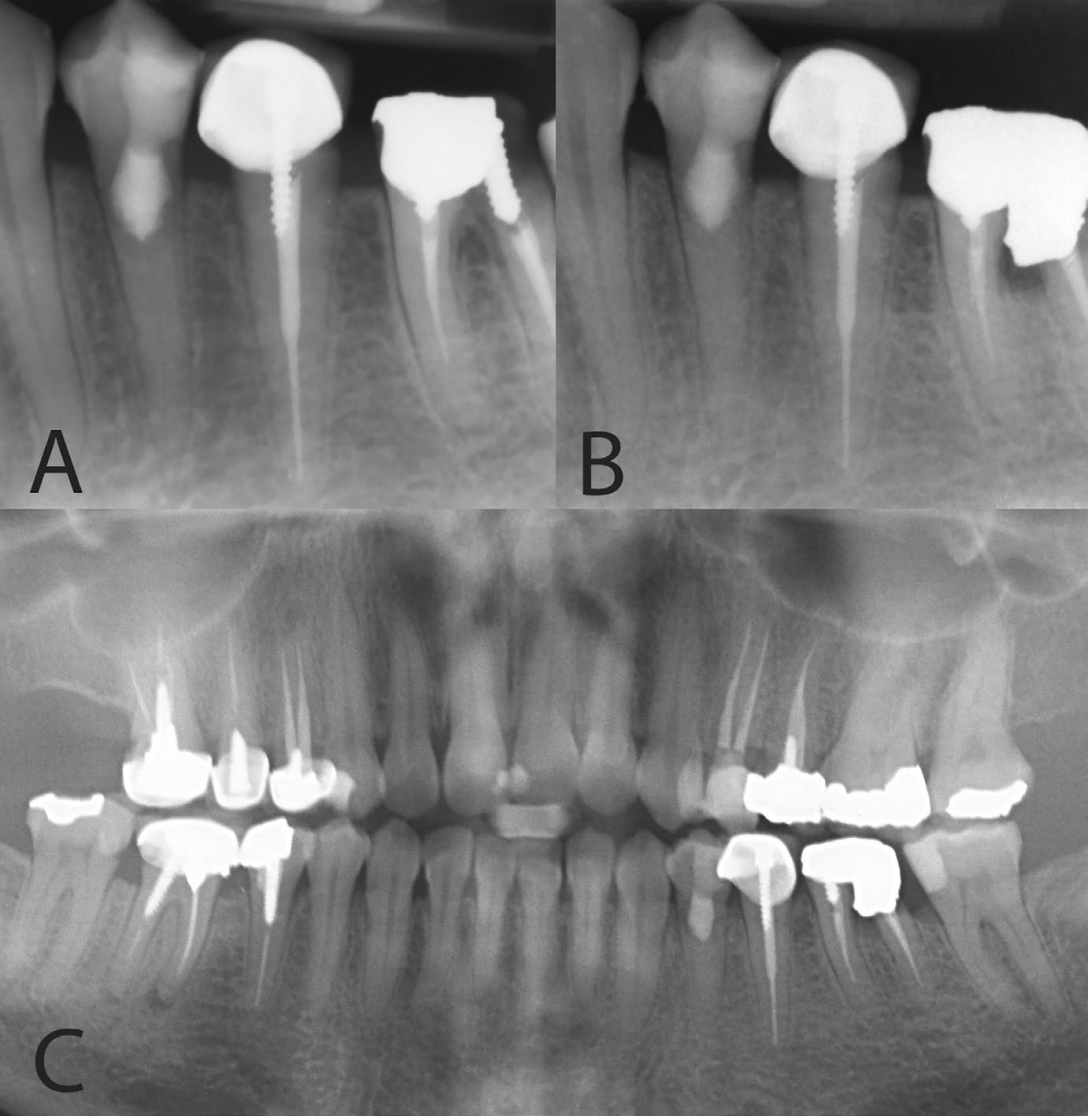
Treatment and follow‐up images of tooth #34. (A) Immediate postoperative periapical radiograph showing successful pulpotomy and proper sealing of the resorptive defect with CEM cement. (B) Two‐year post‐treatment radiograph revealing arrested resorption with a healthy periapical area of tooth #34. The periapical radiograph confirms the effectiveness of the treatment in halting further resorption. (C) OPG at the 2‐year recall, showing no signs of recurrence or additional resorption in the affected tooth. The radiograph highlights the stability of the treated tooth and surrounding structures.
